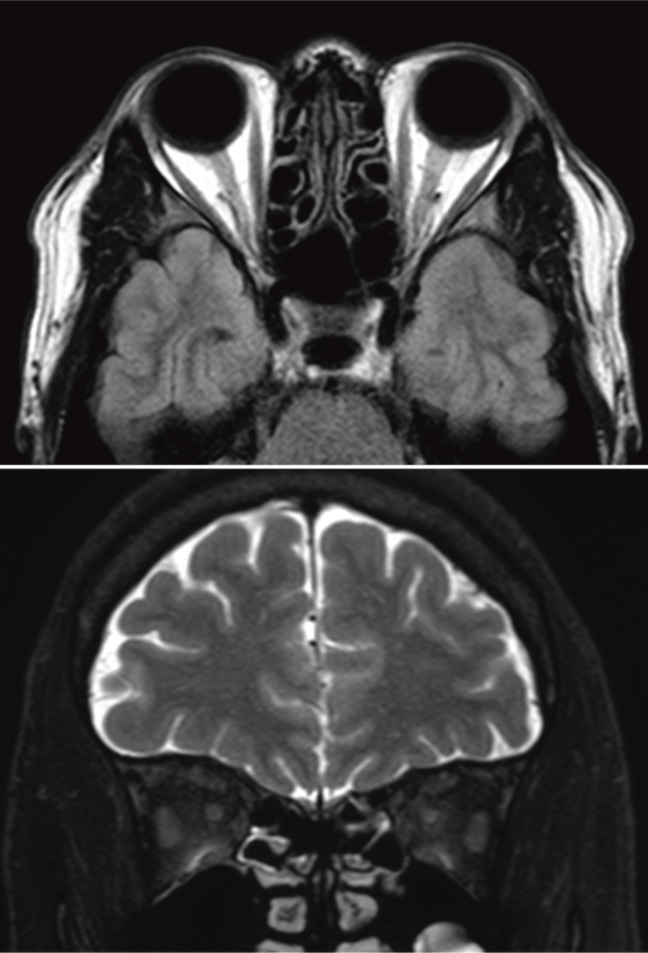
Brain and orbital magnetic resonance images obtained urgently during the initial visit
